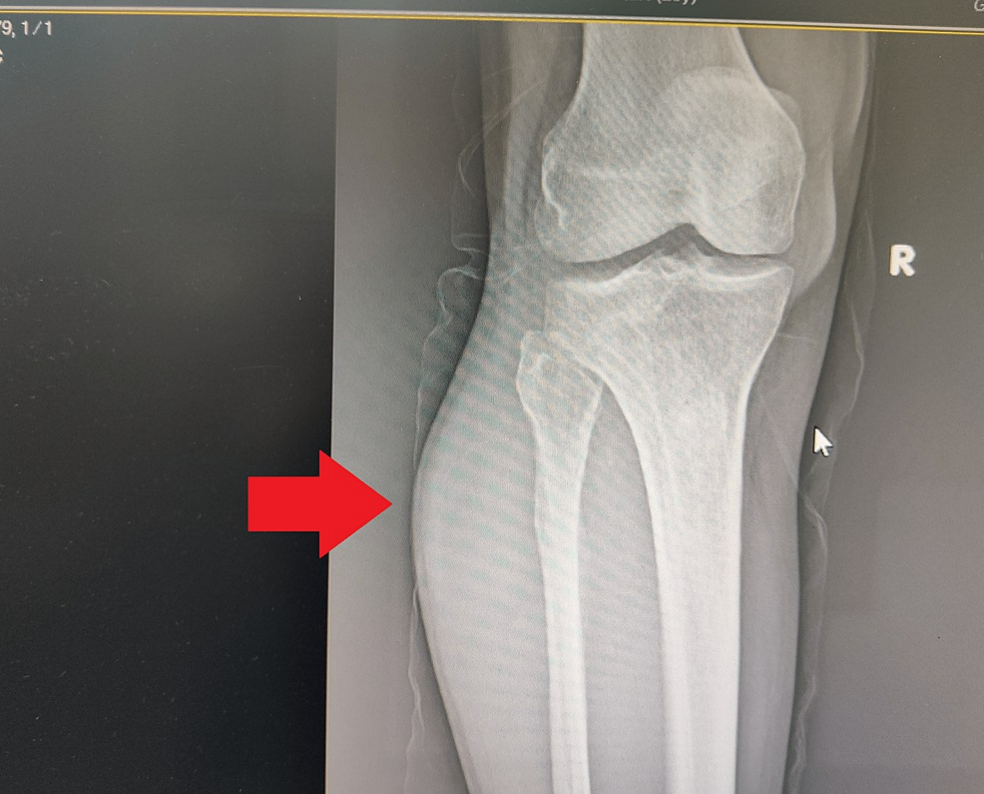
Anteroposterior plain radiograph of the right knee joint demonstrates the bulging of the lateral side of the proximal tibia (red arrow).
